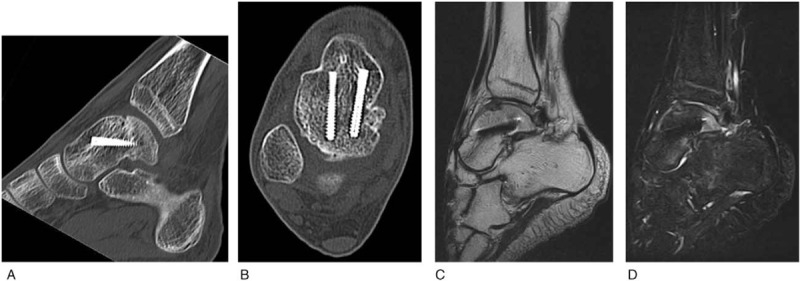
Computed tomography (CT) and magnetic resonance imaging (MRI) at 1 year after the surgery. (A) Sagittal image of CT. (B) Axial image of CT. (C) T1-weighted image of MRI. (D) T2-weighted short-tau invasion recovery image of MRI. The talus fracture has healed and no signs of avascular necrosis or arthrosis are seen.
